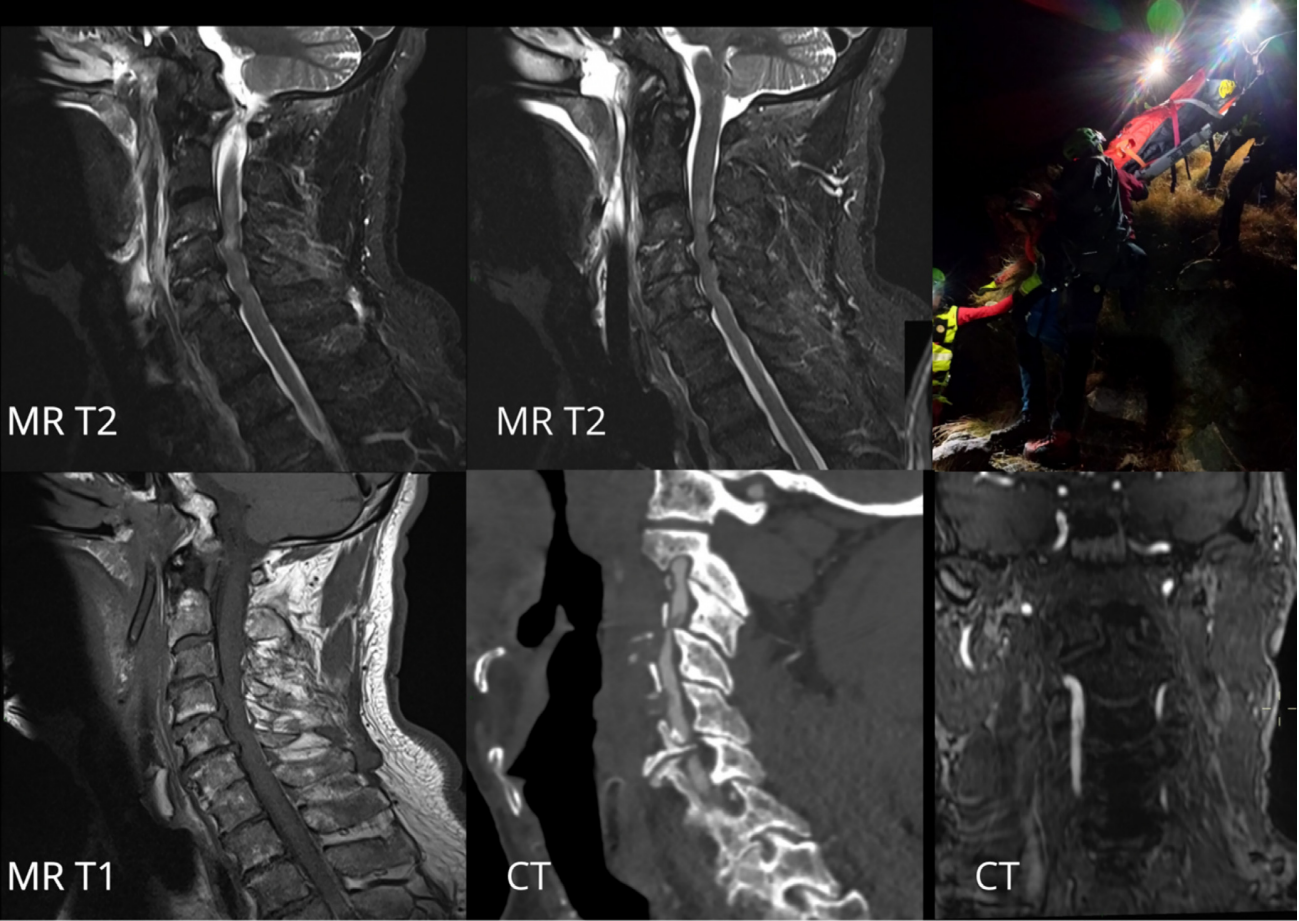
Computed tomography (CT) scan and a magnetic resonance imaging images showing a traumatic spinal cord injury (SCI) at the C2–C4 level, associated with post-traumatic ischemic lesions in the cerebellum secondary to right vertebral artery thrombosis. MR T1 = Magnetic Resonance Imaging, T1-weighted sequence; MR T2 = Magnetic Resonance Imaging, T2-weighted sequence; CT = Computed Tomography.
